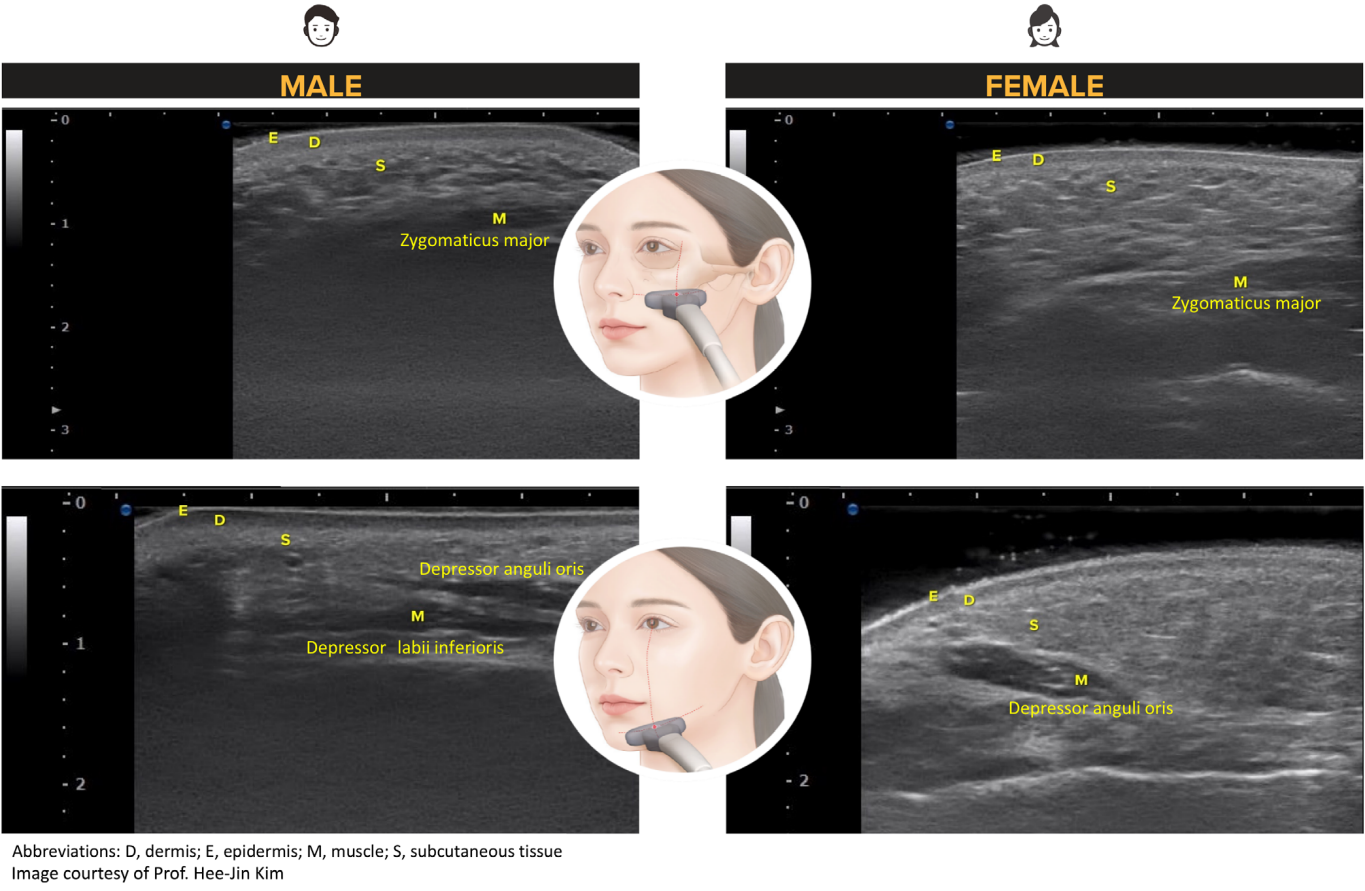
Ultrasound images depicting differences in the thickness of skin layers between male and female patients.
